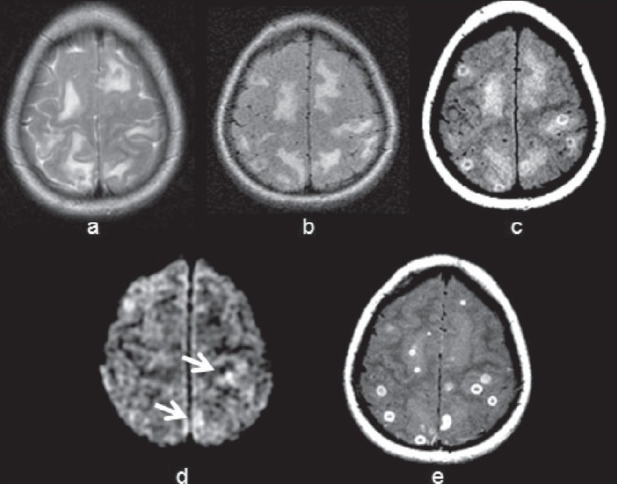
Multiple tuberculomas with perilesional edema exhibiting better definition of the wall on T1W magnetization transfer (MT) imaging in a 20-year-old man. T2W (a) and fluid-attenuated inversion recovery (b) images show areas of central hypointensity surrounded by edema. T1W MT image (c) shows conspicuous hyperintense walls in all lesions. DWI image (d) shows restriction in the core of some of the lesions (arrows). (e) Postcontrast T1W MT image shows ring as well as disc enhancement of all lesions.
